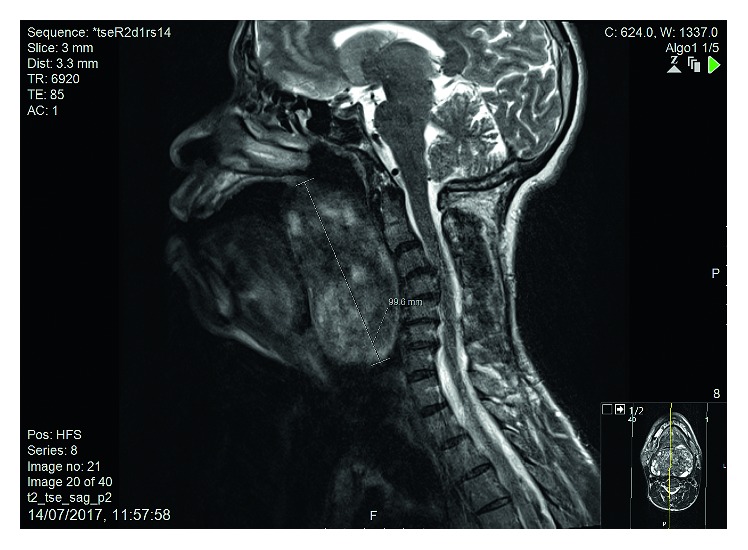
Sagittal T2-weighted MRI image demonstrating the size of the tumour in craniocaudal dimension.
